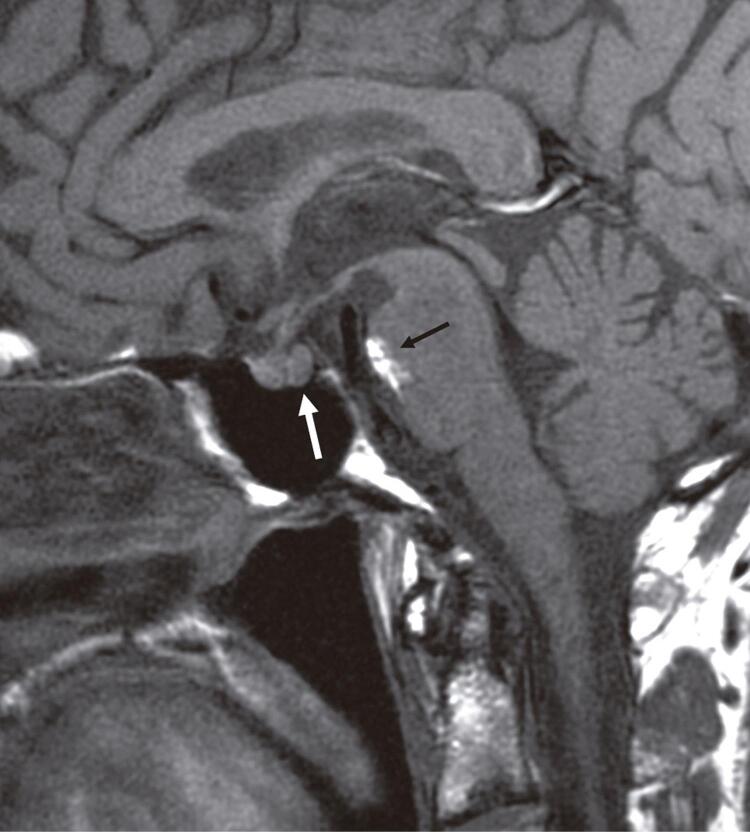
T1-weighted magnetic resonance sagittal image shows absence of high signal intensity of the posterior pituitary lobe (white arrow). In the anterior pons, note a lesion consistent with dermoid cyst (black arrow), as an incidental finding.
